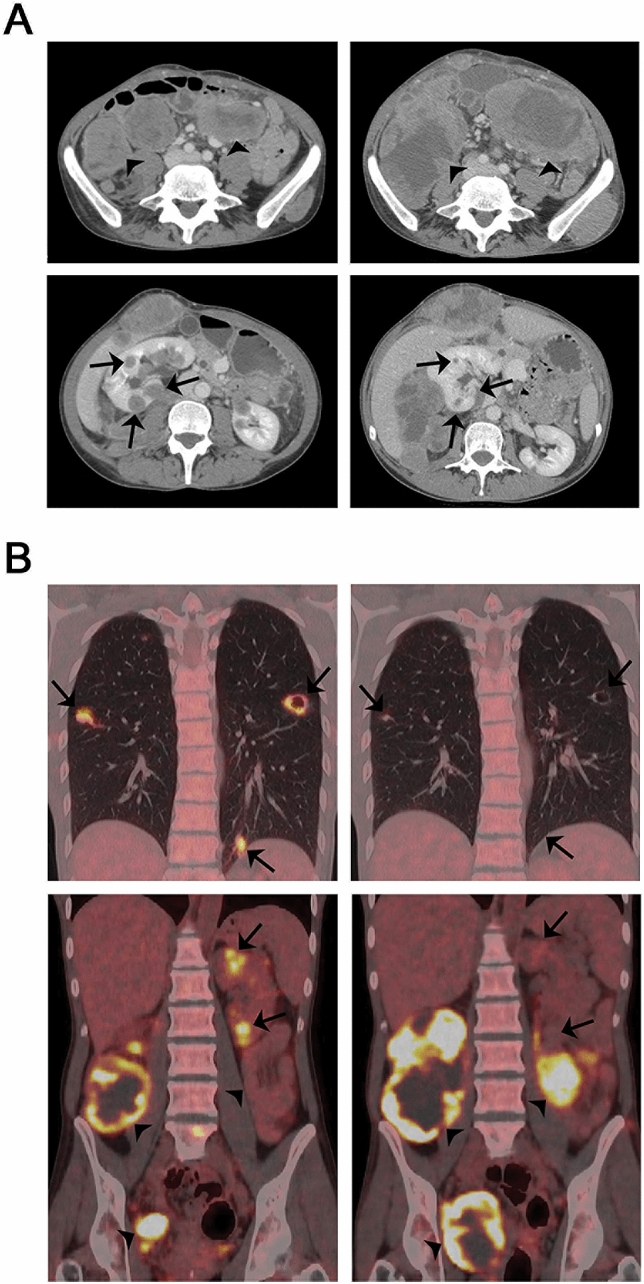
Baseline images and response evaluation after treatment with pembrolizumab in study patient 12 (PROMO-12). a CT images and b 18F-FDG PET/CT images at baseline (left panel) and after two cycles of pembrolizumab (right panel). Arrows indicate reduced uptake of 18F-FDG and tumor size in lung and kidney metastases, and arrowheads indicate progressive abdominal and pelvic metastases
